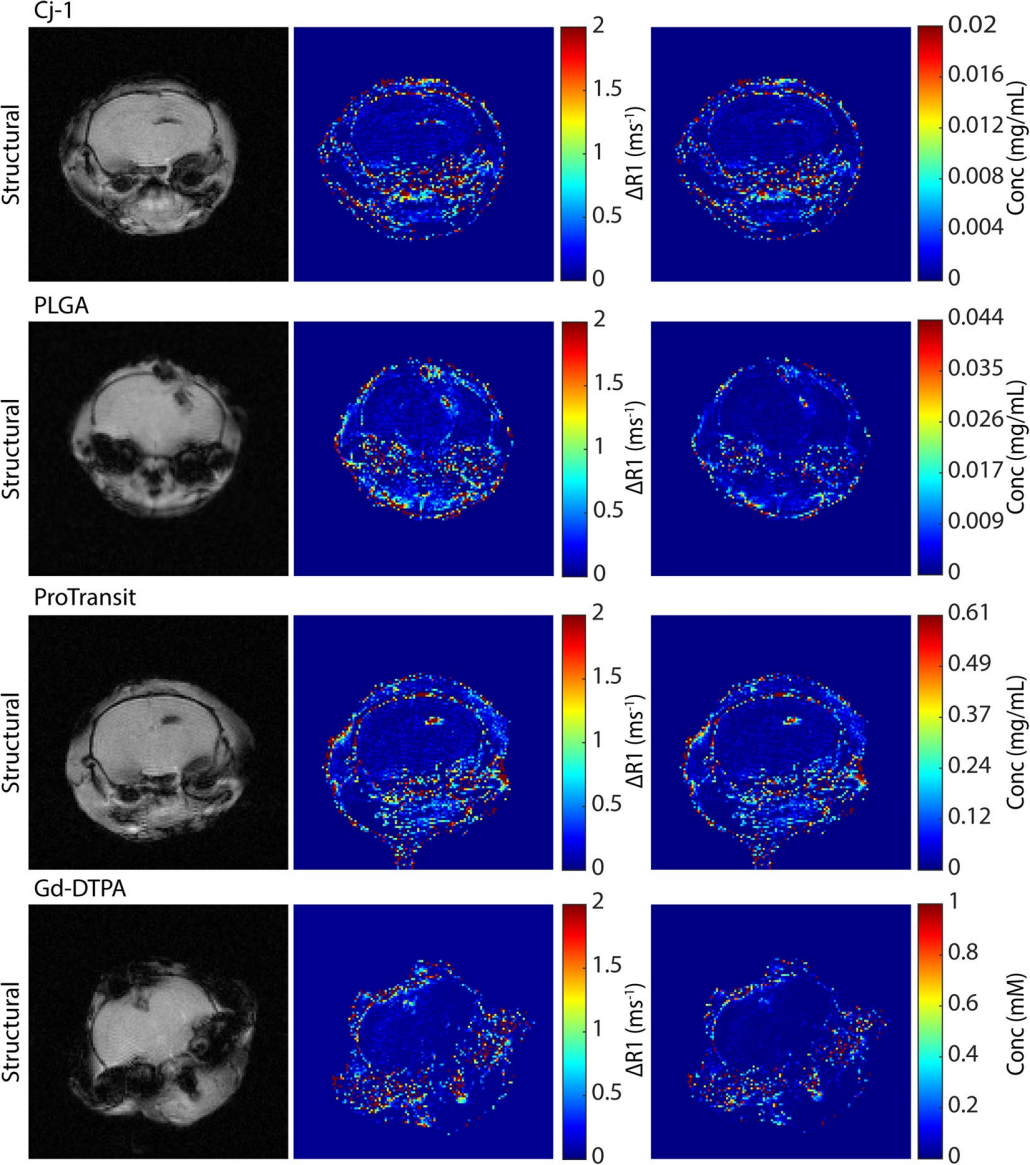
Structural MRI, ∆R_1_, and concentration maps for the three NPs and Gd-DTPA. Structural images show injury, dark area near top of brain, ∆R_1_ and concentration maps show change in R_1_ and NP concentration between final and initial scans. Highest ∆R_1_ and concentration values correspond to the injured regions in the structural images. Concentration units for Gd-DTPA have been displayed as mM and for NPs as mg/mL in an attempt to maintain use of units most familiar for these particles.
